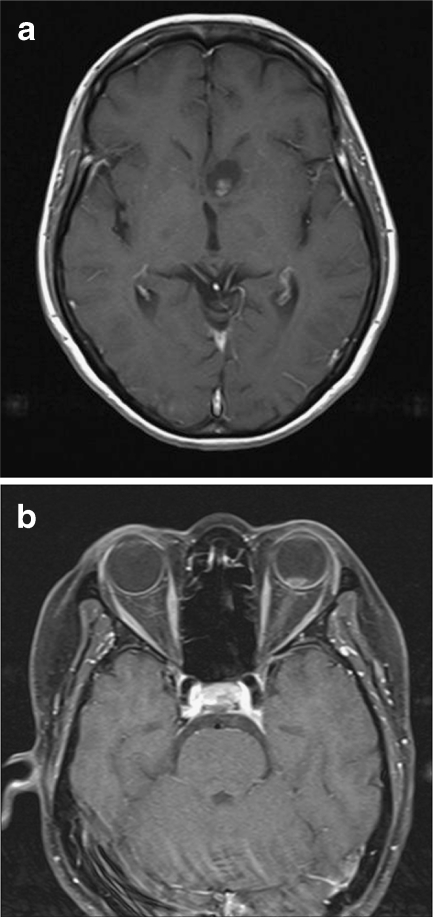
Gadolinium-enhanced brain and orbital magnetic resonance images show a cystic mass with a solid internal enhancing component in left caudate nucleus (a) and an enhanced solid mass in the left eye over the optic nerve head (b)
